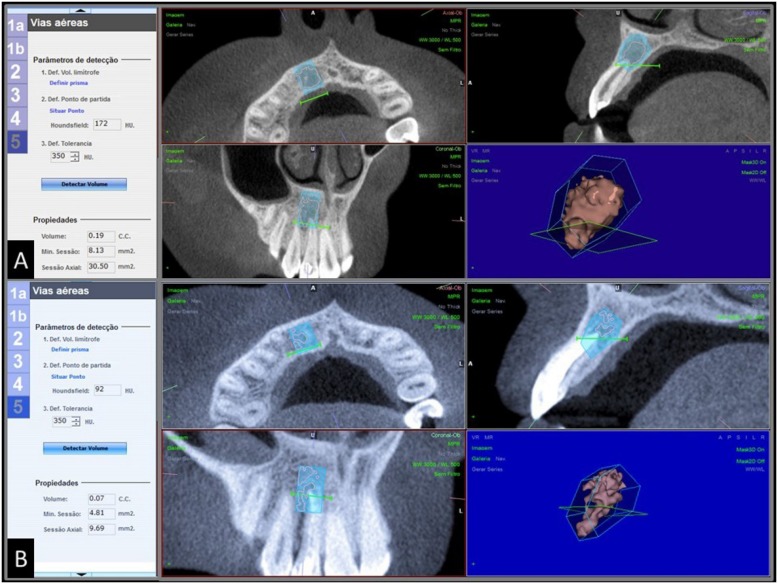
DICOM data of (A) preoperative and (B) 18-month follow-up analysis transferred to NEMOTEC^®^ software (Madrid, Spain), and 3D reconstruction of periapical lesion
